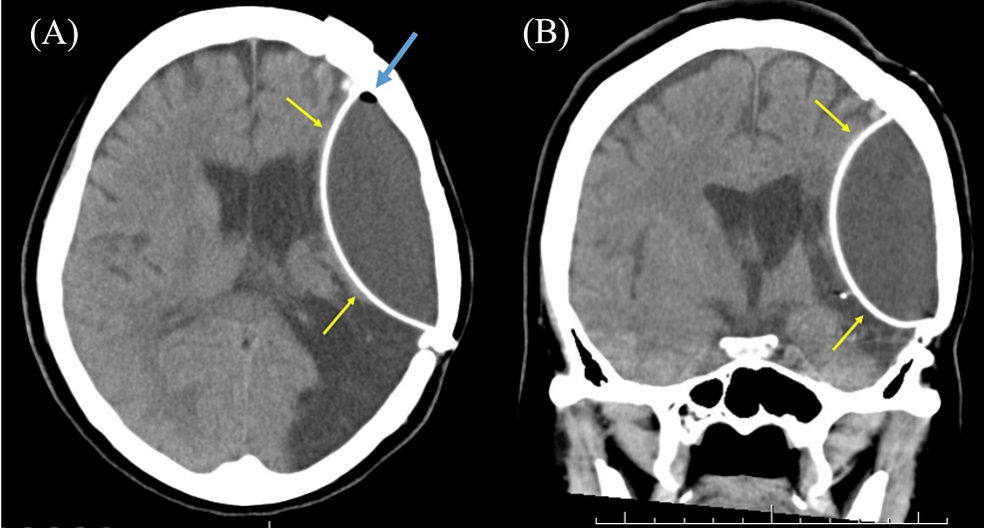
The result of brain computed tomography (CT). Brain CT showing a double skull sign (DSS) in the right hemisphere (yellow arrowheads). In addition, a small amount of air is noted inside the DSS (blue arrowhead). (A) Axial section; (B) coronal section.
